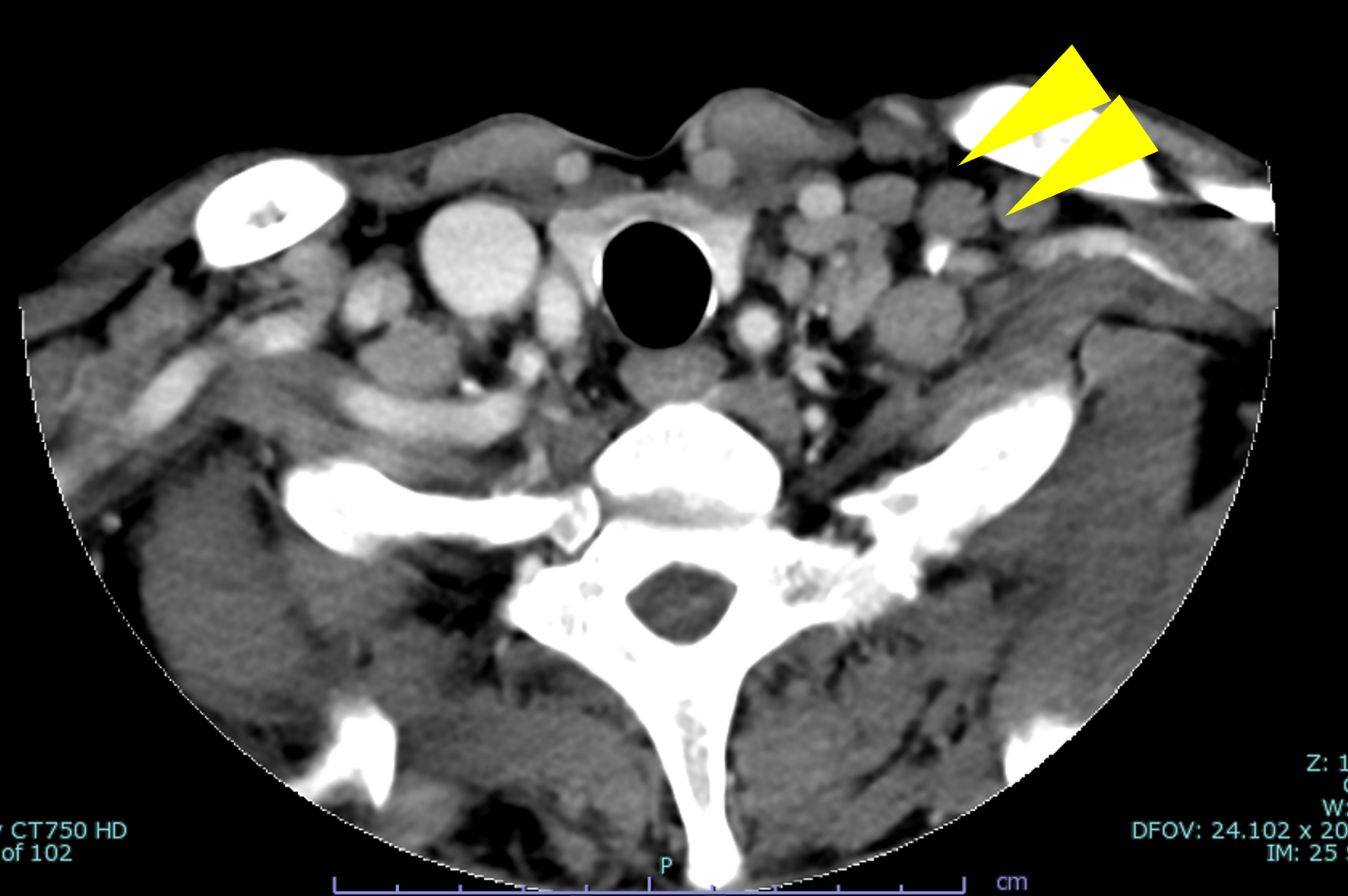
A computed tomography scan demonstrates swelling of supraclavicular lymph nodes (yellow arrow).
